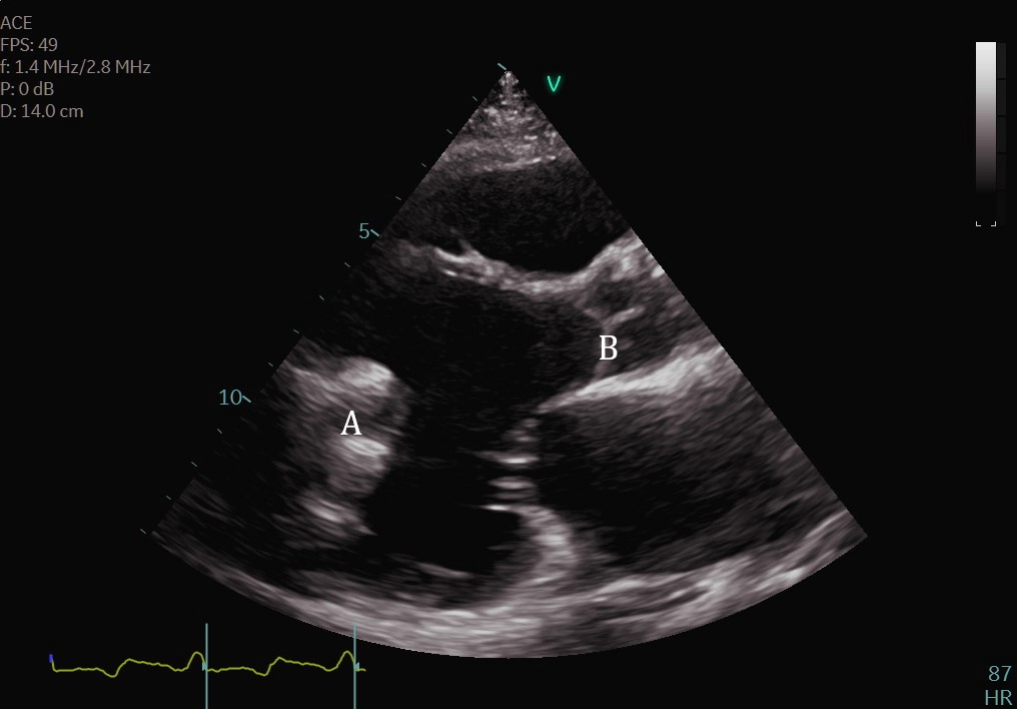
Transthoracic echocardiogram PLAX image demonstrating inflow
